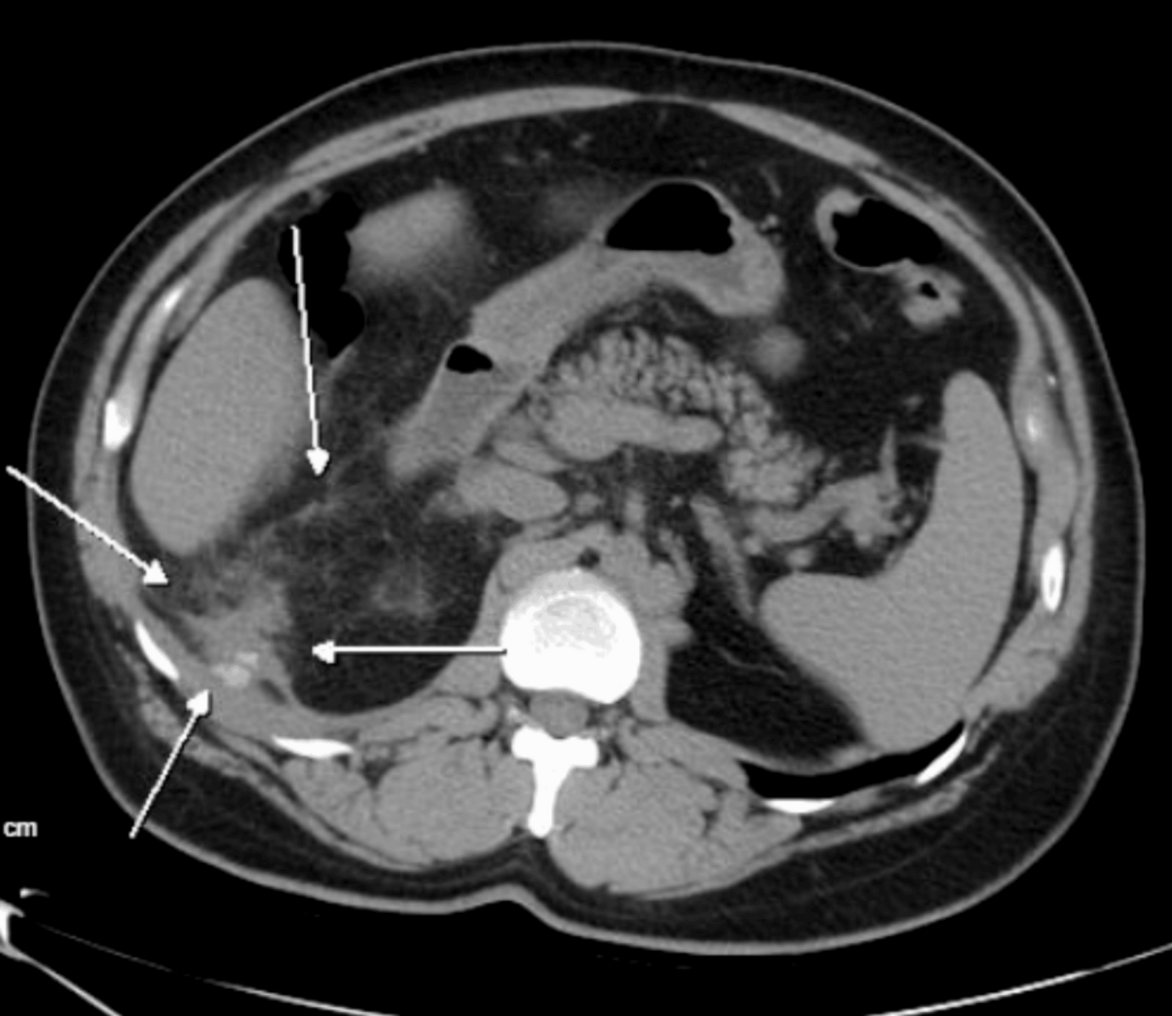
CT scan of the abdomen and pelvis demonstrating two hyperdense foci within the hyperdense area along the inferior margin of the right hepatic lobe. CT, computed tomography
